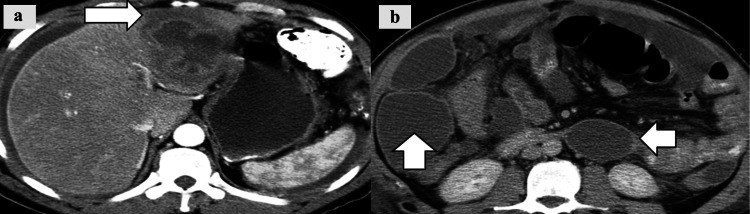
Intraperitoneal abscess. a: CT image shows hepatic abscess (white arrow); b: CT image shows multiple intraperitoneal fluid collections (white arrows) secondary to liver abscess rupture.
